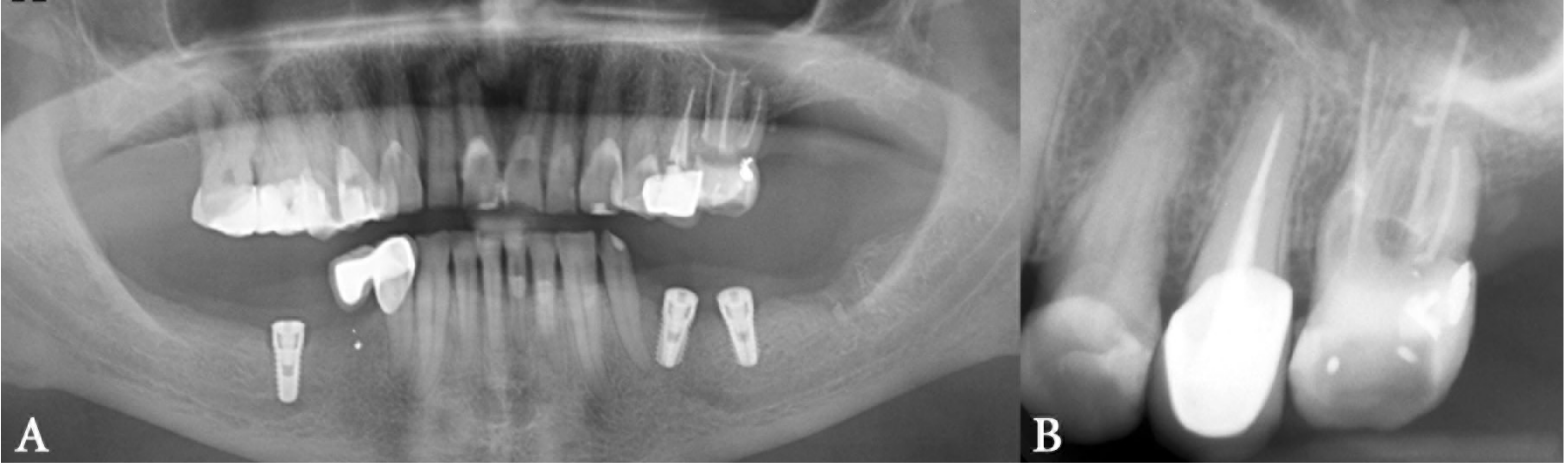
A) *Panoramic image of the patient with the chief complain being dull pain in the left side of the face; tooth #26 was sensitive to percussion. *B) *Periapical view; endodontic treatment of tooth had been done five weeks earlier
